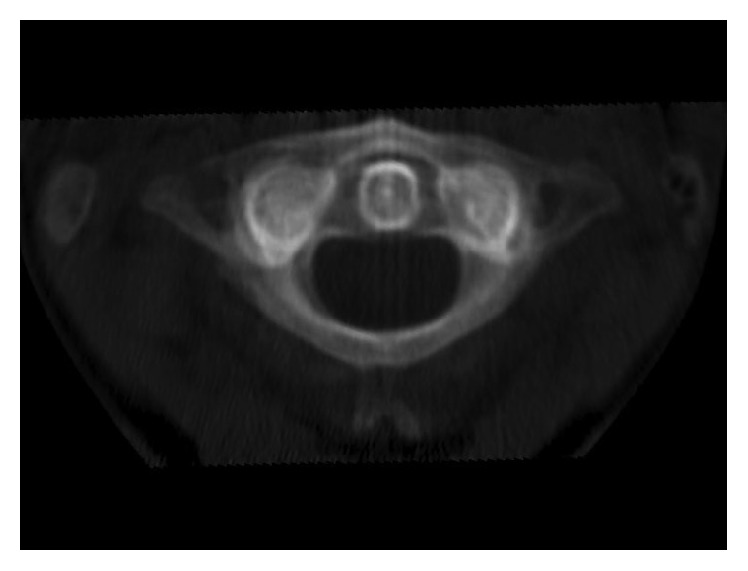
This axial CT scan depicts C1-C2 after reduction, CT-MIP (maximum intensity projection).
